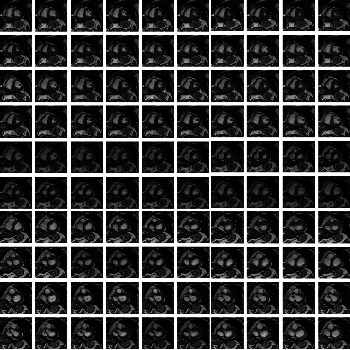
Depiction of selected frames from a 3D MRI sequence viewed in the short-axis orientation to demonstrate the application of augmentation technique through random rotation.
